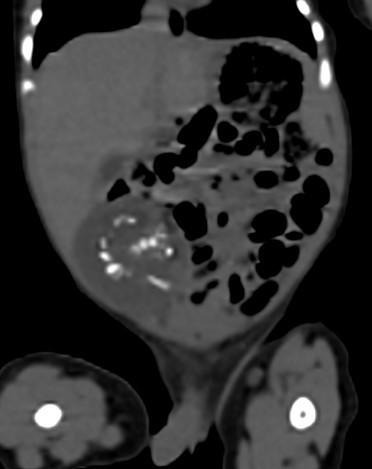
Post-natal CT image showing multiple curvilinear areas bone density structures within the hypodense mass which appear like vertebrae long bones and part of the calvarium.
